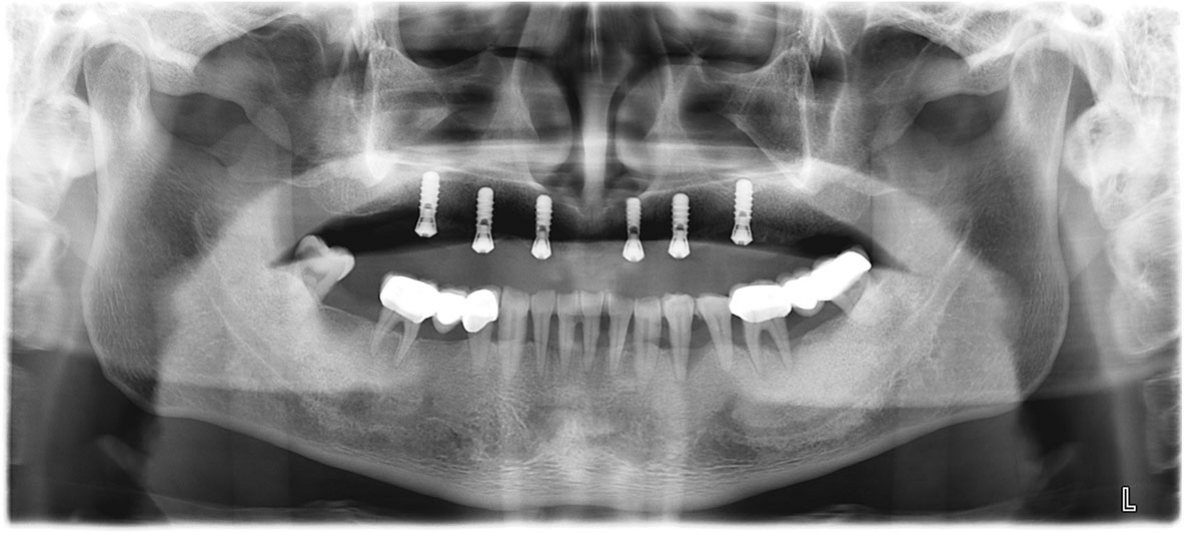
Post-placement panoramic x-ray
